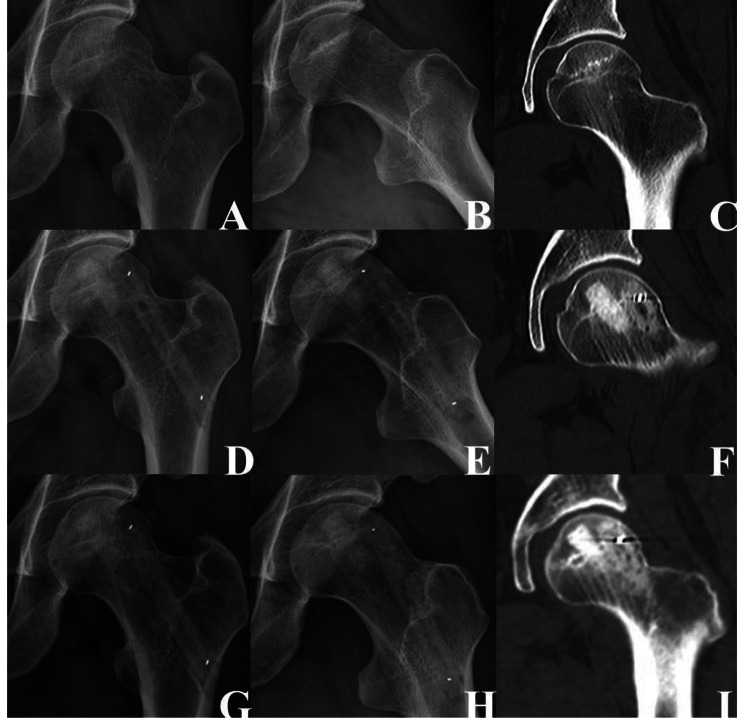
Images of a 50-year-old man who presented with type 2 left nonspecific femoral head necrosis. (A–C) Preoperative radiograph and computed tomography showed that the femoral head was rounded. (D–F) Three days after the operation, radiographs and computed tomography showed the shadow of the support rod and the bone graft. (G–I) Two years after the operation, radiographs and computed tomography showed that the femoral head was round and the bone density had increased
